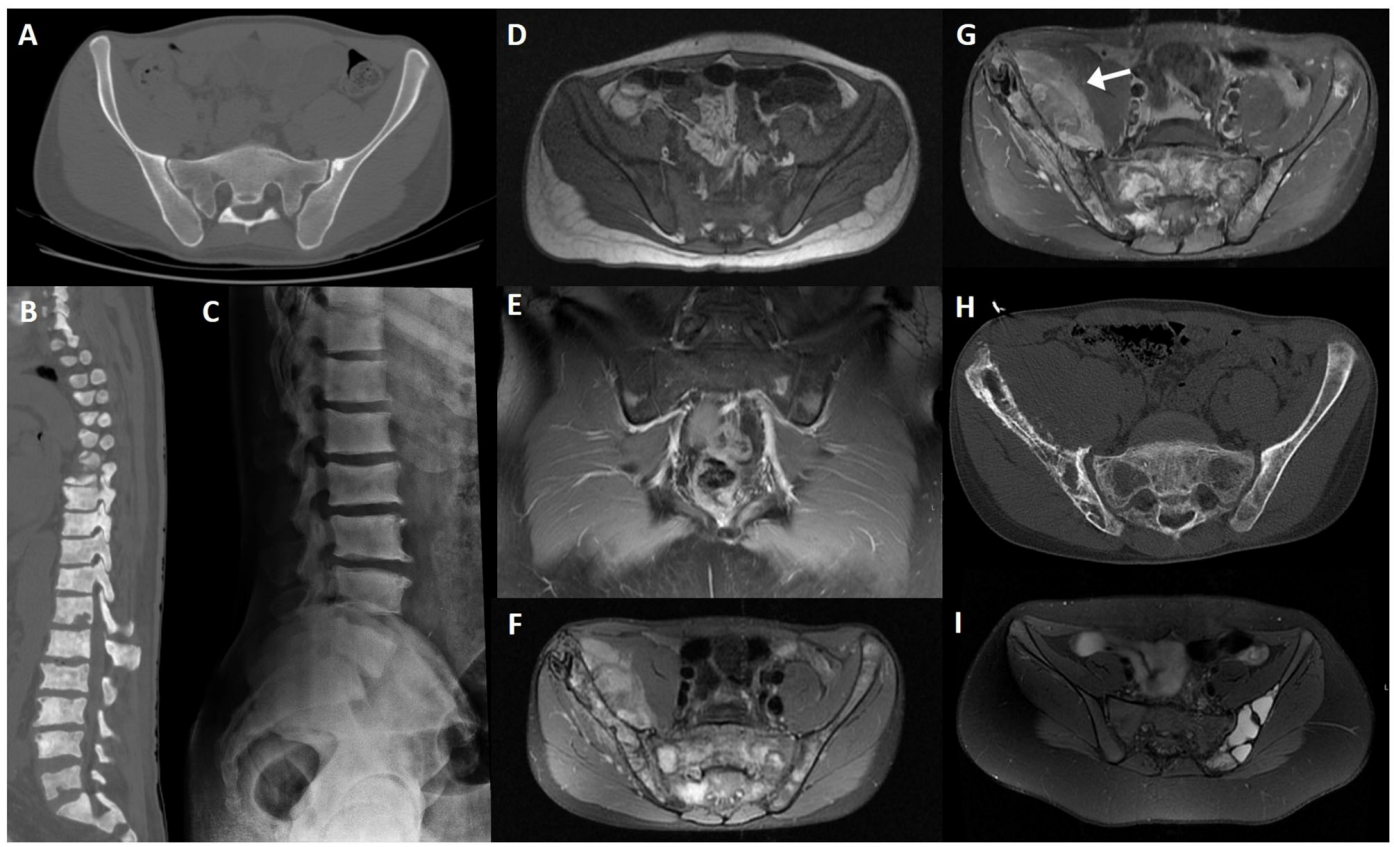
CT axial slice (A) of an iliac bone enostosis mimicking peri-articular sclerosis; CT sagittal reconstruction (B) of the dorsal and lumbar spine in a patient with diffuse osteoblastic metastasis due to prostate cancer; lateral lumbar radiograph of the same patient (C); T1WI axial slice (D); and post-contrast fat-saturated T1WI coronal slice (E) of a patient with leukemic infiltration of the sacrum and iliac bones, showing diffuse bone marrow T1 hypointensity due to tumoral infiltration and multifocal patchy uptake, respectively; fat-saturated PD (F), post-contrast fat-saturated T1WI (G) MRI and CT axial slice (H) of a 18-year-old male patient with Ewing sarcoma; fat-saturated PD (I) MRI sequence of an aneurysmatic bone cyst of the left iliac bone.
